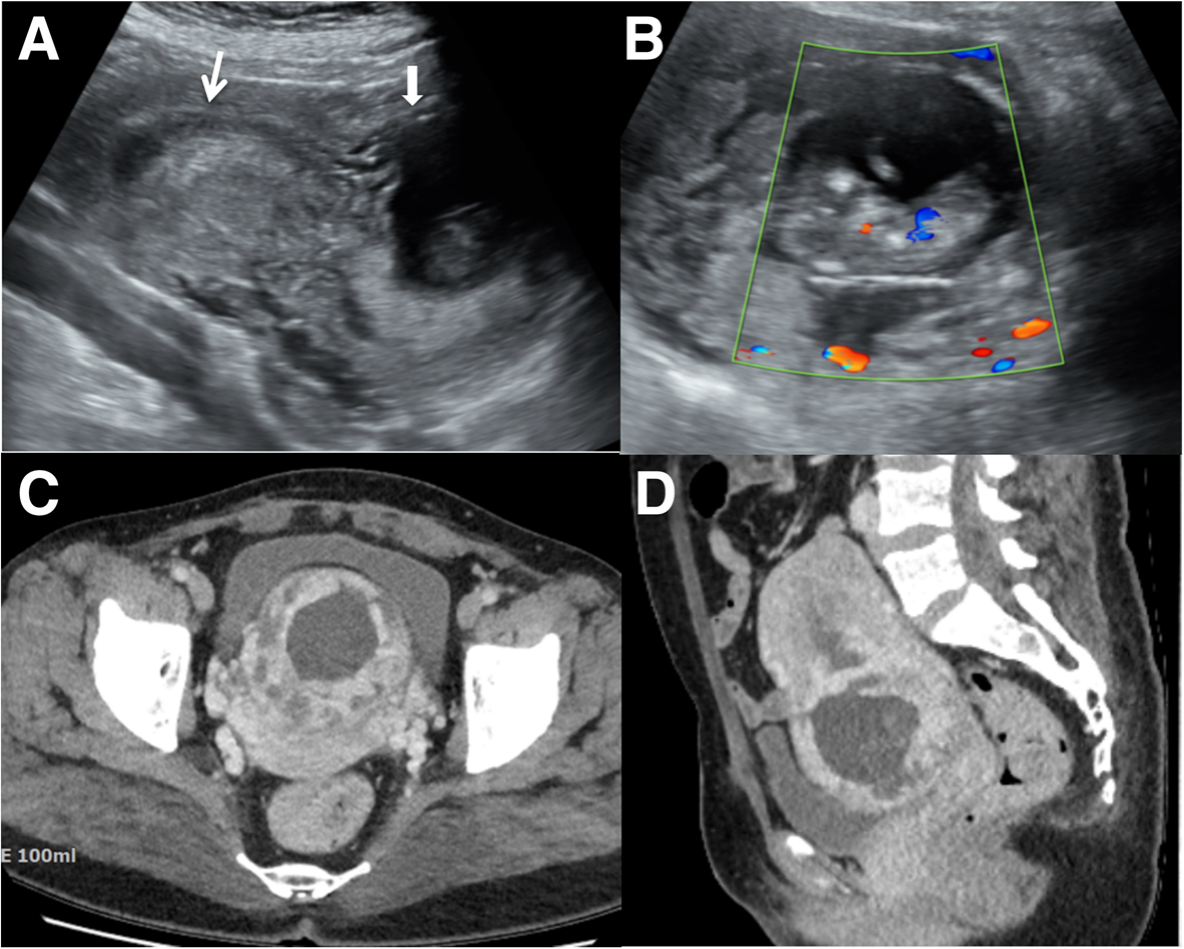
The initial transabdominal ultrasonography images show a gestational sac (bold arrow) located anteriorly in the lower uterine cavity with an empty uterine endometrial cavity (narrow arrow) (a). The color/power Doppler images depict a hyperechoic rim of choriodecidual reaction with excessive vascularity (b). The computed tomography image shows an intrauterine gestational sac in the lower uterine segment bulging through the anterior uterine wall at the site of the cesarean scar without invasion of the urinary bladder (c, d)
